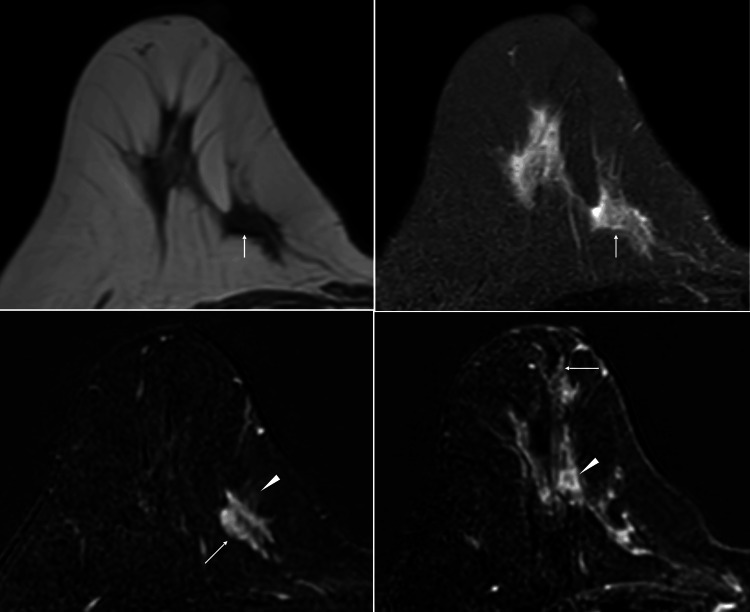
MRI findings MRI of the tumor showed low signals on T1-weighted images (A, arrow), slightly high signals on fat-suppressed T2-weighted images (B, arrow), fast enhancement (C, arrow), enhancement of the cancer cell infiltration areas in the subcutaneous fat (C, arrowhead), and a daughter nodule (D, arrowhead) with presumed ductal spread (D, arrow) on dynamic studies.
